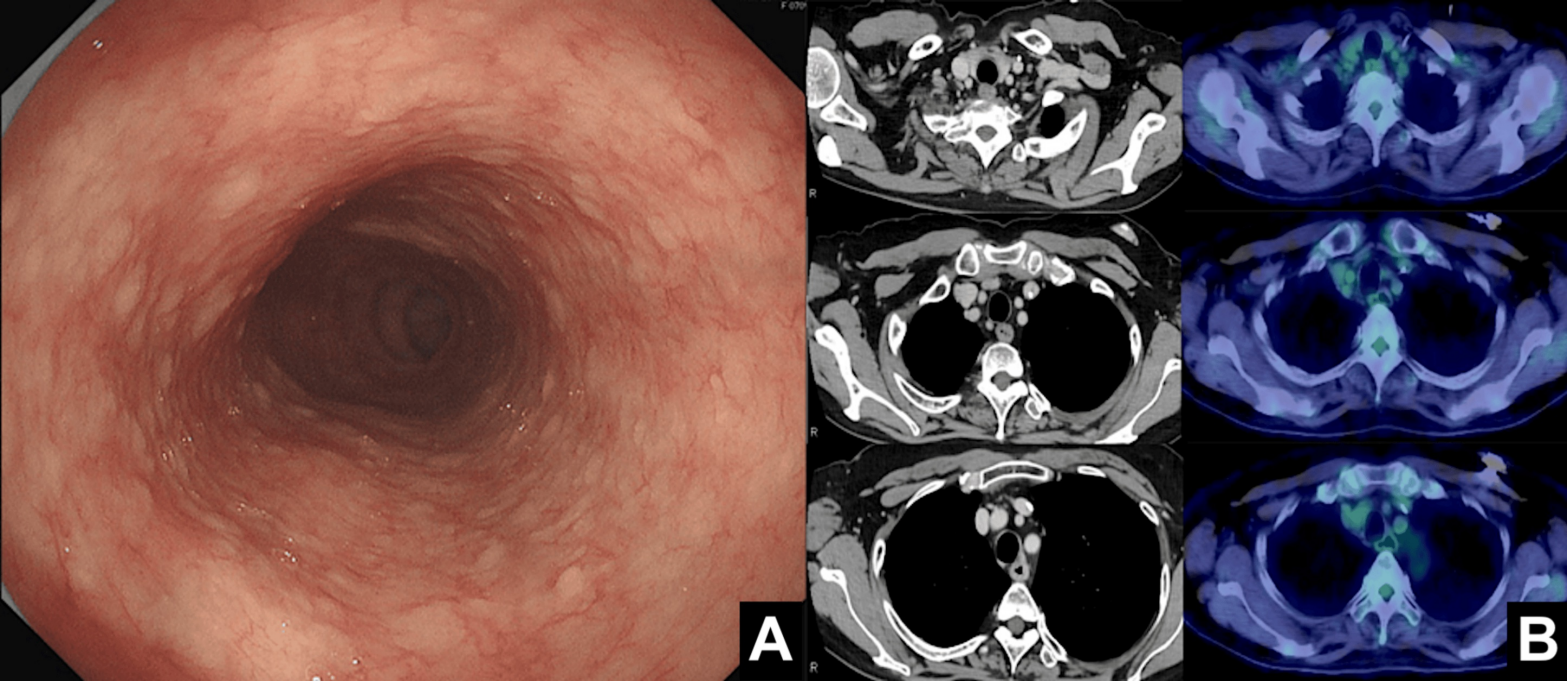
Post-treatment imaging findings. (A) Endoscopic image after chemotherapy showing a reduction in the size of the protruded lesion, indicating a favorable response to induction therapy. (B) Post-treatment CT (left) and PET-CT (right) images showing resolution of the lymph node enlargement and FDG uptake in the same areas, suggesting a favorable response to induction chemotherapy.
